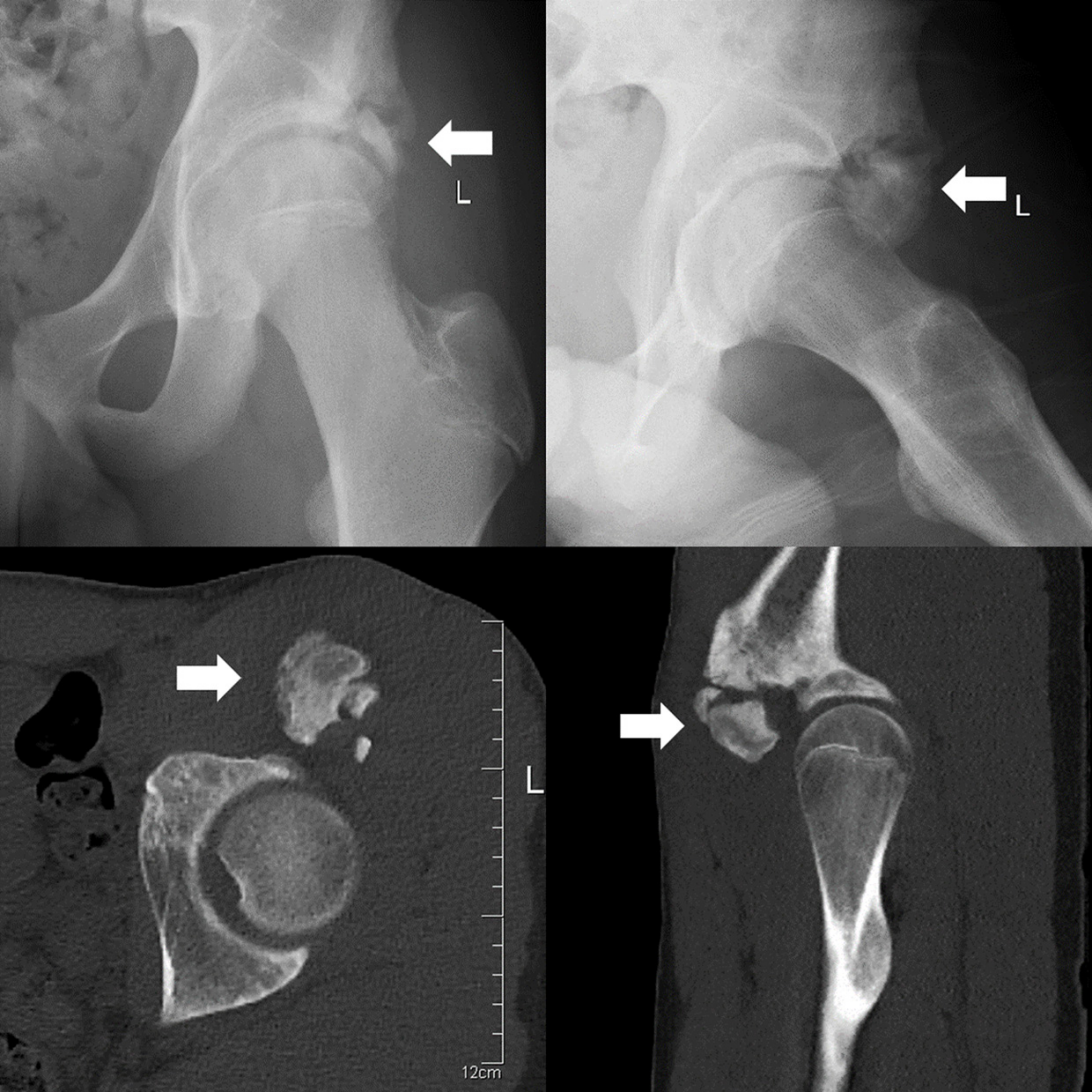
Pre-operative radiograph and CT scan. Arrows show the area of heterotopic ossification that occurred in the rectus femoris muscle near the origin of the anterior inferior iliac spine.
